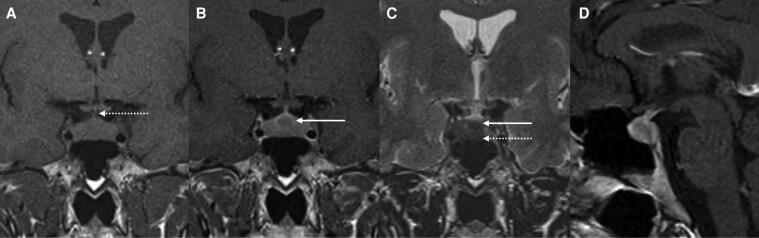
Sellar magnetic resonance imaging on A, coronal T1; B, coronal T1 post contrast; C, coronal T2; and D, sagittal T1 post contrast showing a symmetrical pituitary gland enlargement with convex superior margin (dotted arrow in A), and marked T2 hypointensity (dotted arrow in C), which was initially considered to be a pituitary adenoma because of a misinterpreted central nodule (white arrow in B and C).
